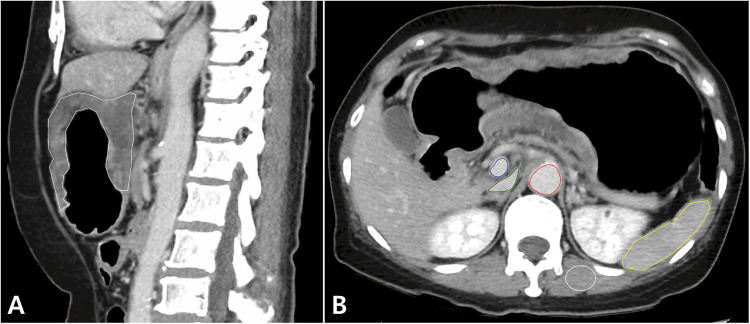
Representative case of measurement of CT attenuation. A. Measurement of CT attenuation value of gastric cancer, sagittal portal venous phase image. B. Measurement of CT attenuation values of the main portal vein (blue), inferior vena cava (green), aorta (red), spleen (yellow), and back muscle (white), axial portal venous phase image.
